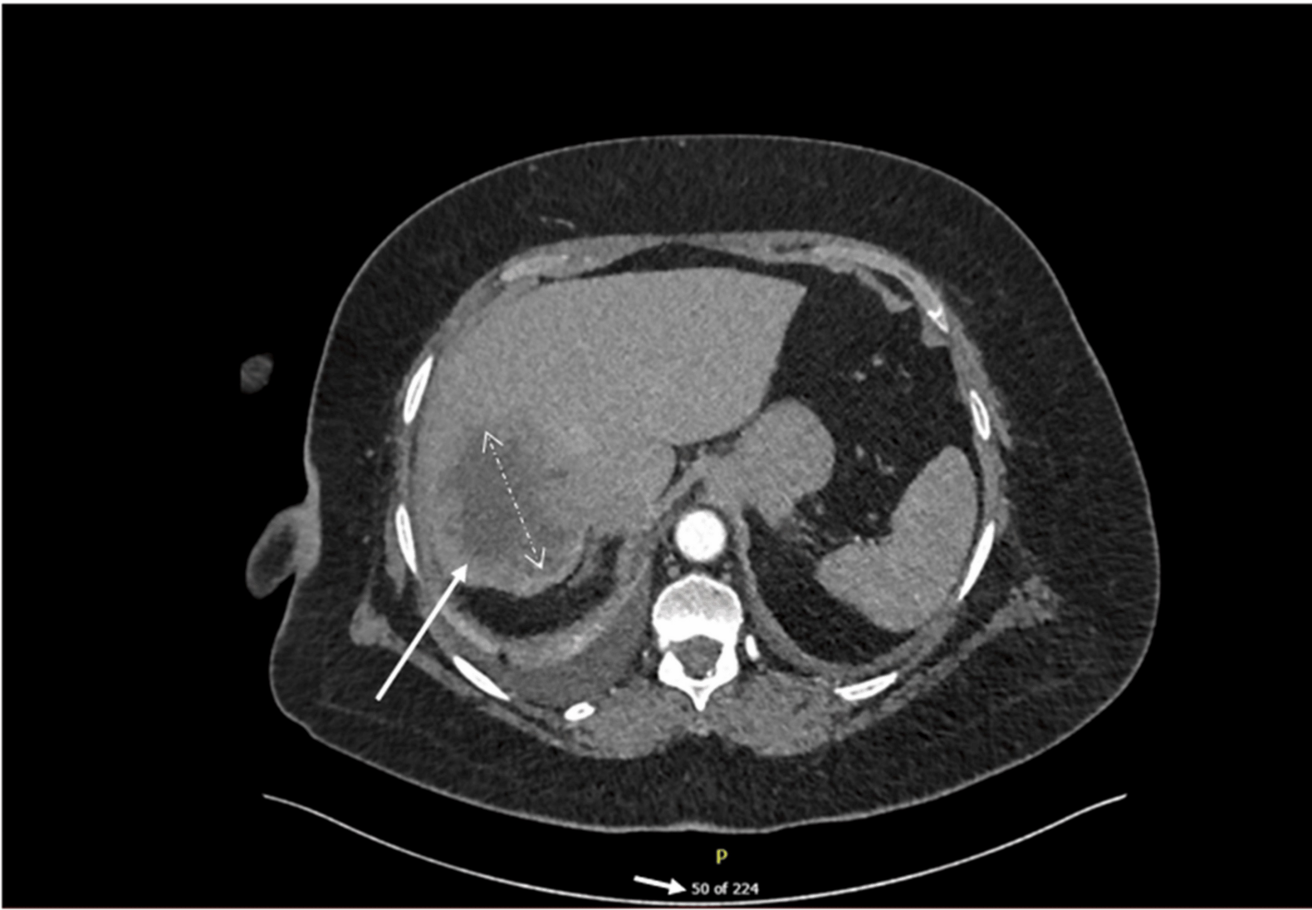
CTPA image showing hepatic lesion CTPA: Computed tomography pulmonary angiography There is a large hepatic dome lesion (white arrow) measuring about 8 cm in diameter, showing peripheral irregular enhancement with central hypodensity. There are multiple flecks of calcifications within the lesion.
